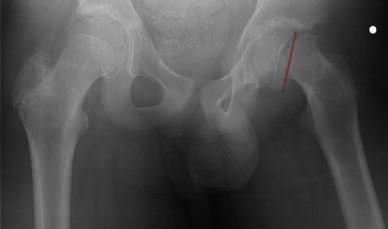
A line is drawn across the corners of the epiphysis on an anteroposterior (AP) radiograph of the pelvis
